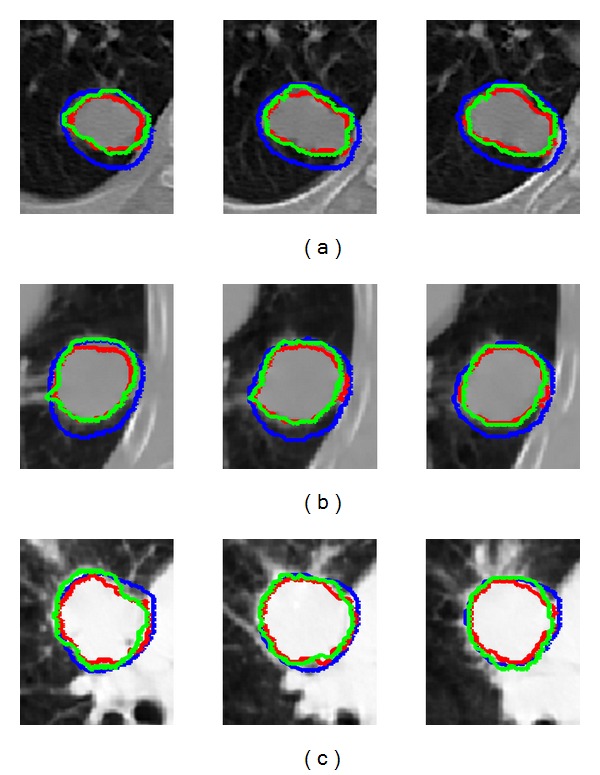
GTVs in axial CT slices of patient (a), (b), and (c). GTVs in blue are the results with fuzzy MRF method using only PET images and GTVs in red and green are the results with the new method and manual method, respectively, using both PET and CT images.
